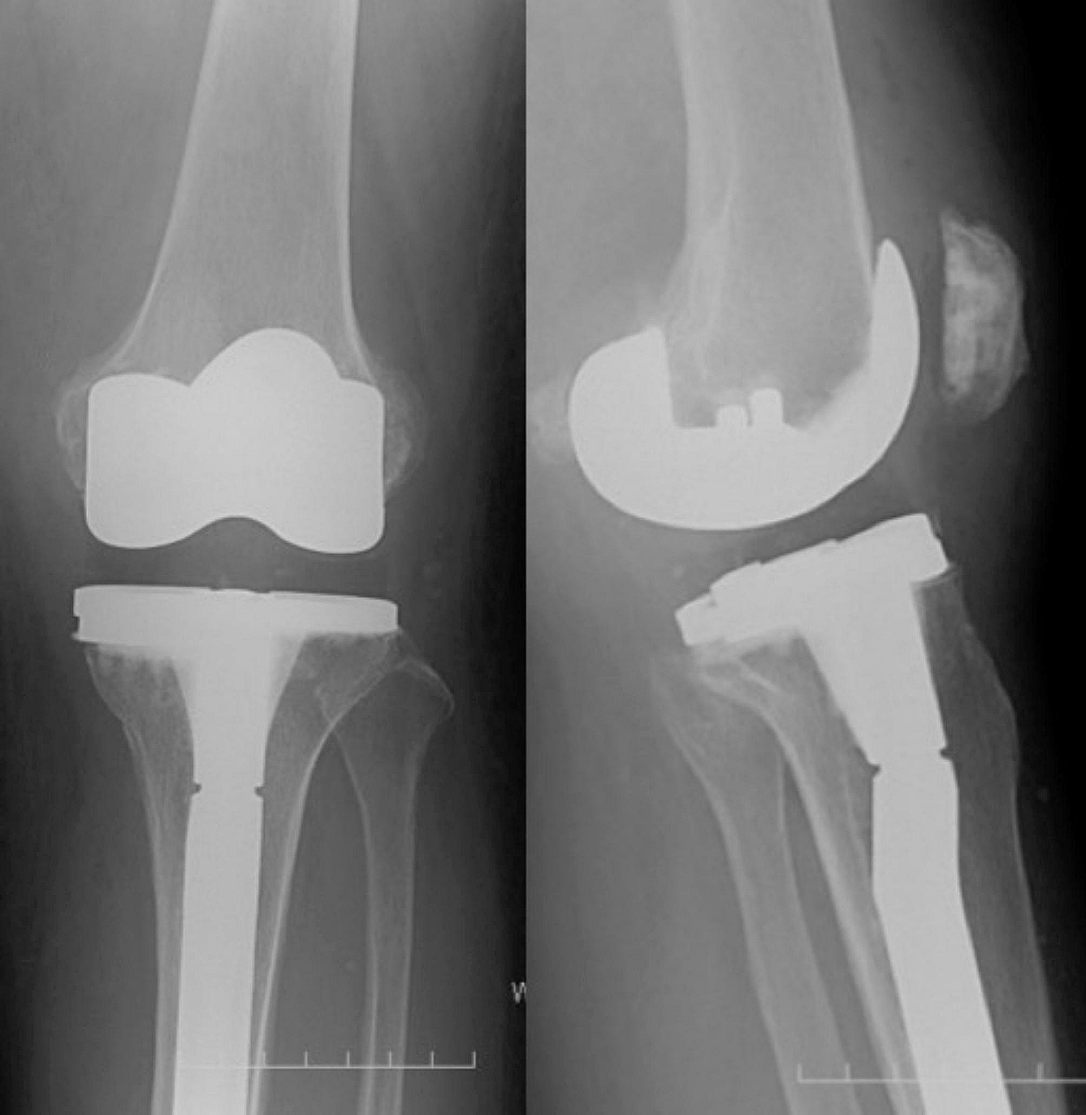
Case 1 Simple X-ray image at the time of the first visit to this hospital AP view & Lateral view
